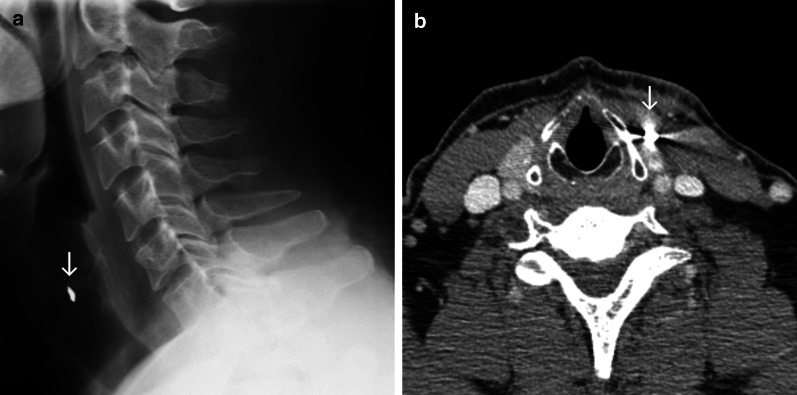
Broken hammer. Lateral radiograph (a) and axial contrast enhanced CT image (b) showing a radiopaque/hyperdense structure (arrow) anteromedial to the sternocleidomastoid muscle and lateral to the thyroid cartilage. Streak artefacts in the CT image indicate marked density, a potential clue that the foreign object is metallic
